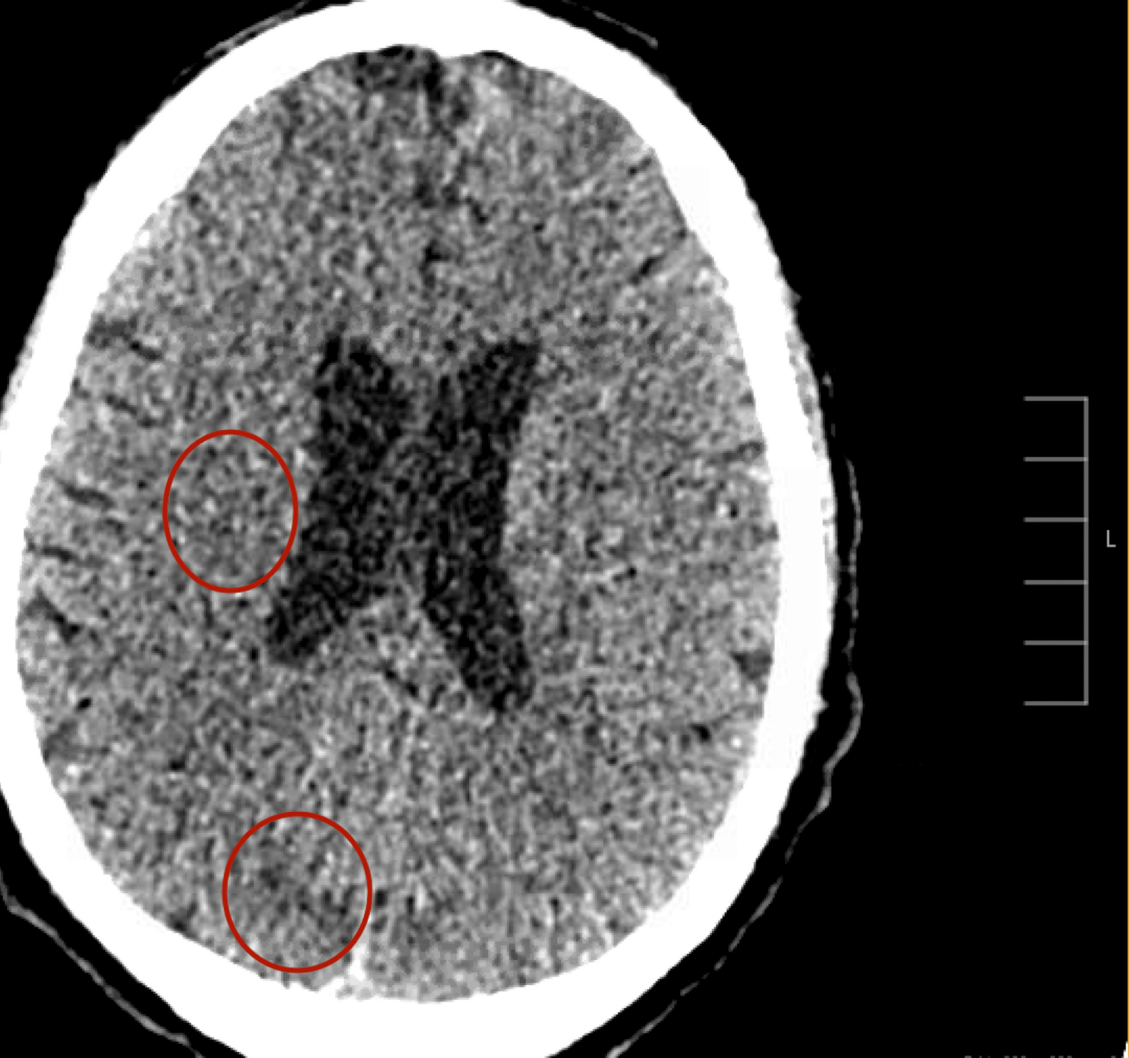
CT head showing acute infarct and early ischemic changes in the right medial parietal and occipital lobes
